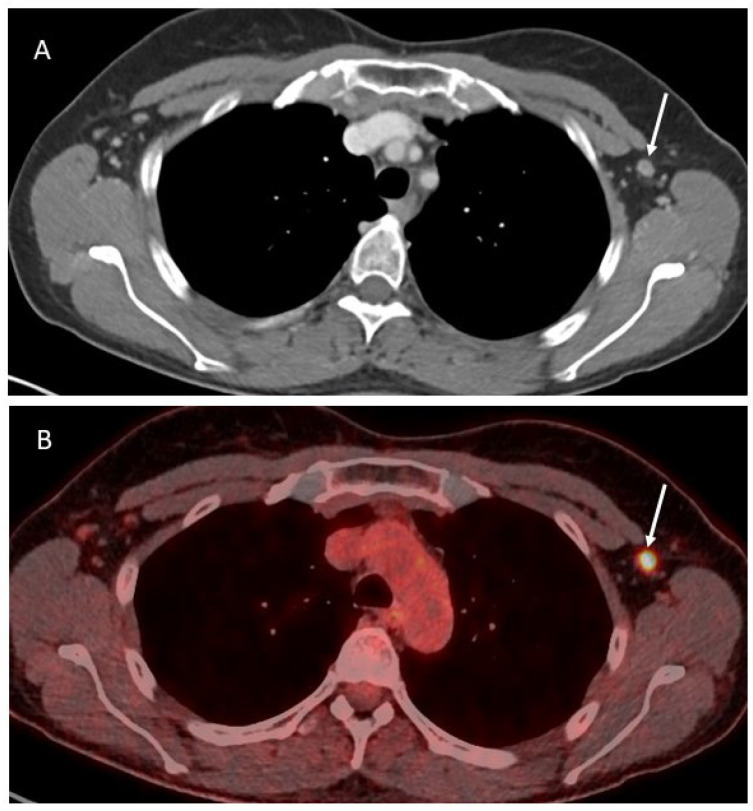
Axial contrast-enhanced CT image (A) showing a slightly enlarged lymph node in the left axilla (white arrow), corresponding to a pathologic activity on the PET-CT (B).
